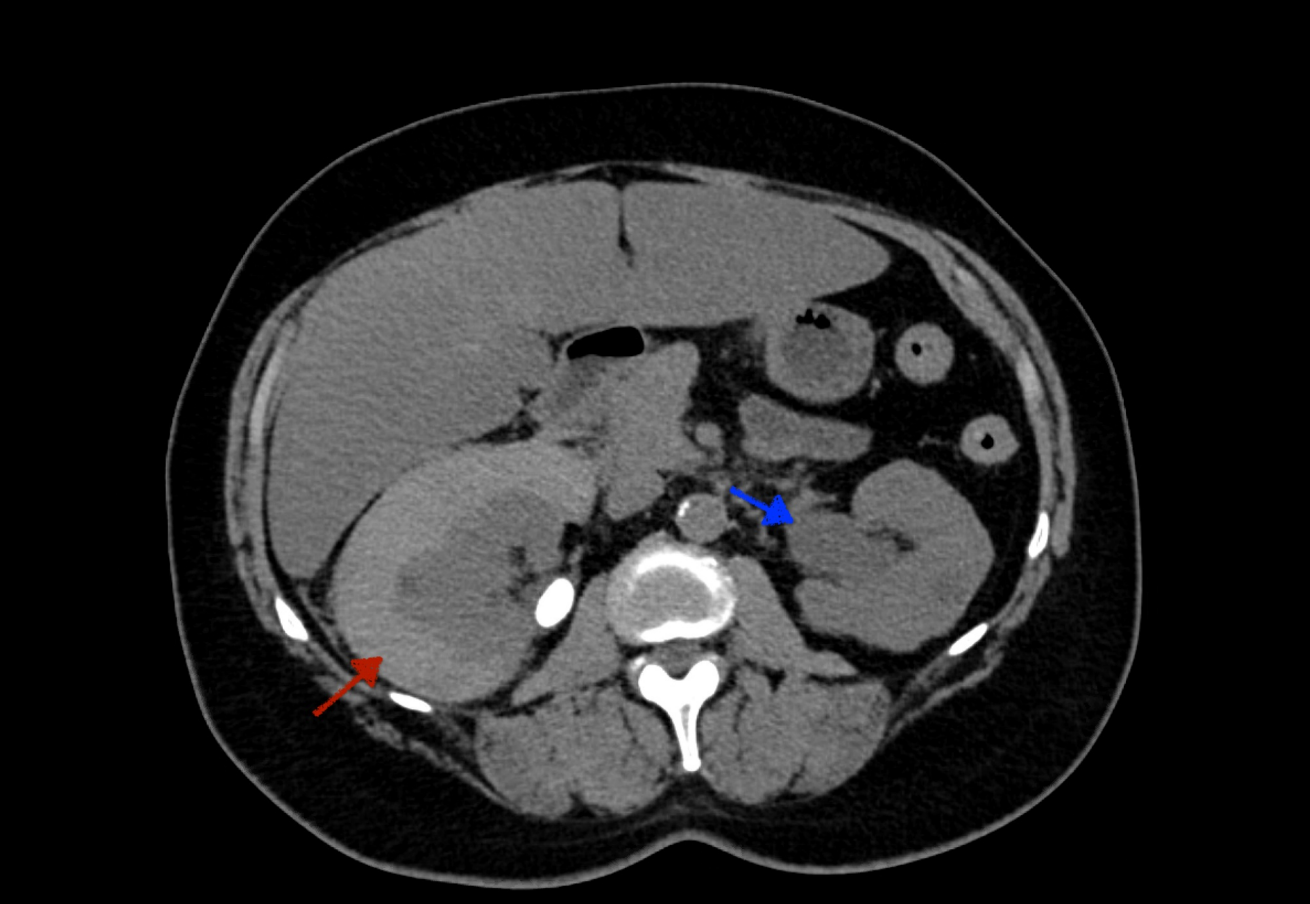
CT Scan A subcapsular hematoma of the right kidney (red arrow) and mild to moderate left hydronephrosis (blue arrow) due to distal ureteral calculus.
